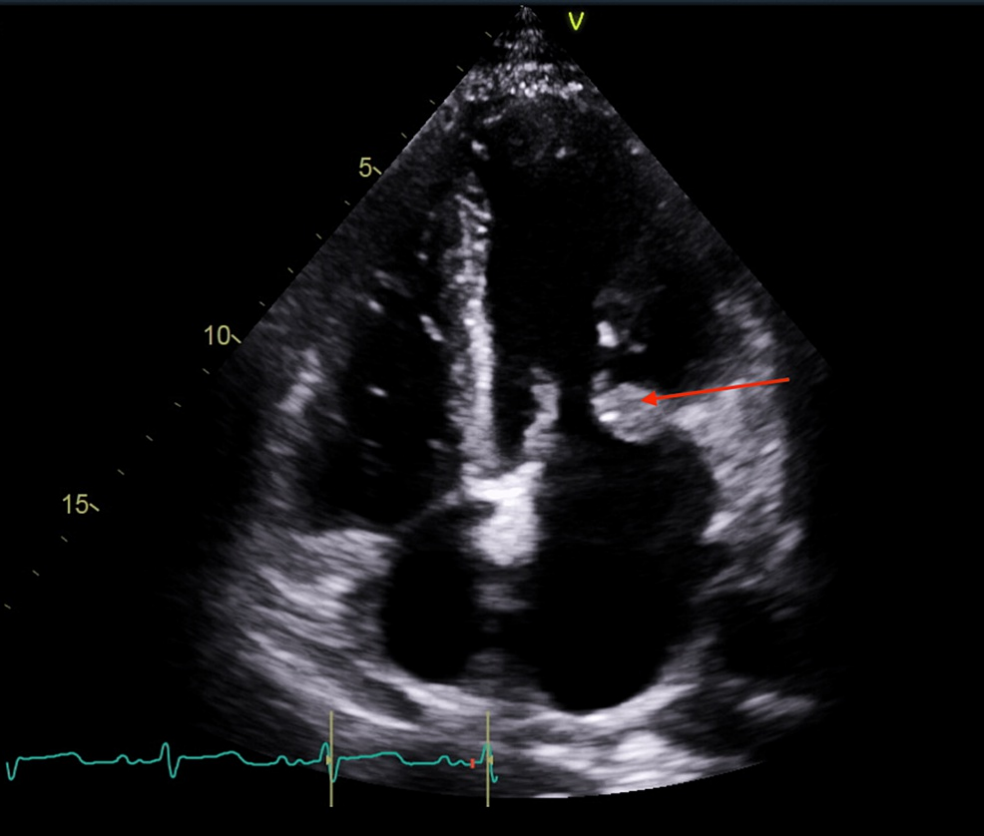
Transthoracic echocardiogram apical four-chamber view. The red arrow indicates 2.0 × 2.6 cm fixed mitral valve vegetation in apical four-chamber view.
